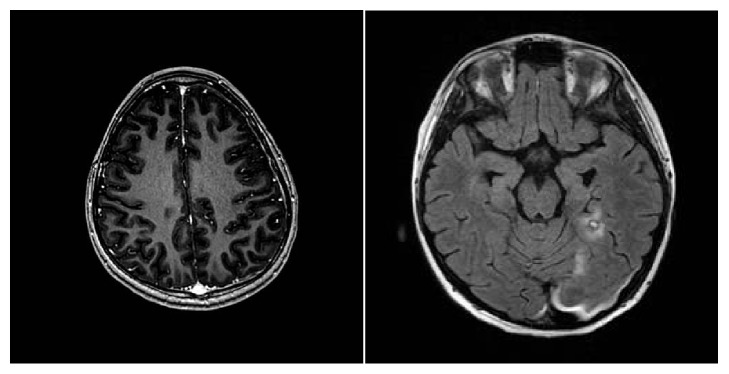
MRI Brain FLAIR images demonstrating multicompartmental hemorrhages and leptomeningeal enhancement.
